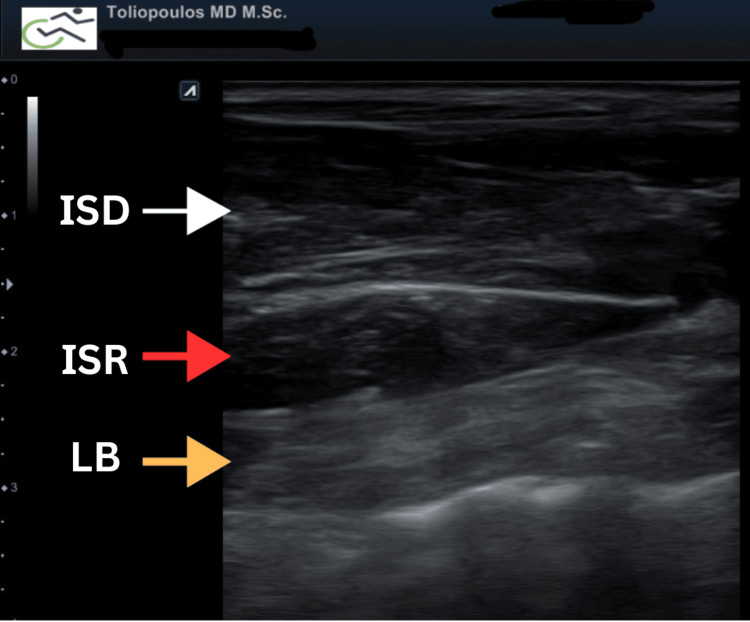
Ultrasound appearance of the hand intrinsic muscles. The white arrow indicates the dorsal interosseous muscle, the red arrow points to the palmar interosseous muscle and the yellow arrow highlights the lumbrical muscle.
